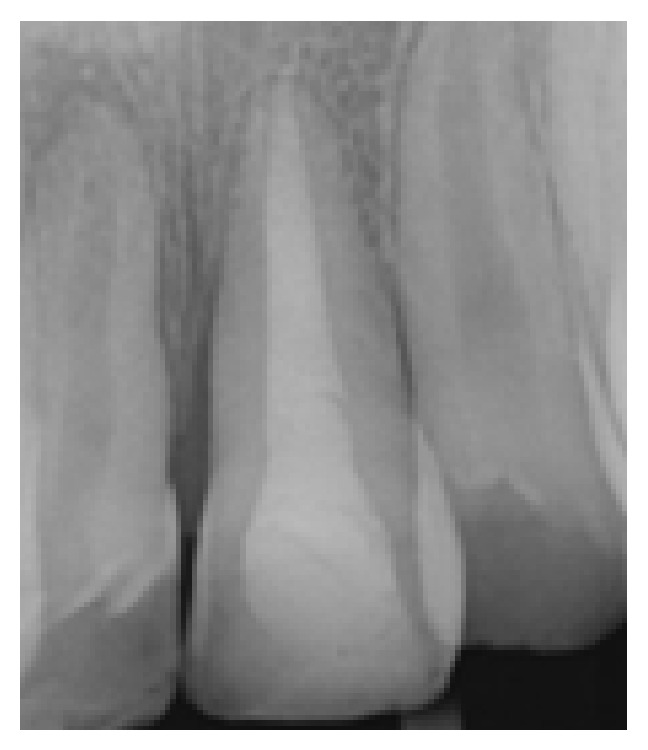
No pathology and resorption, 12-month radiographic examination.
